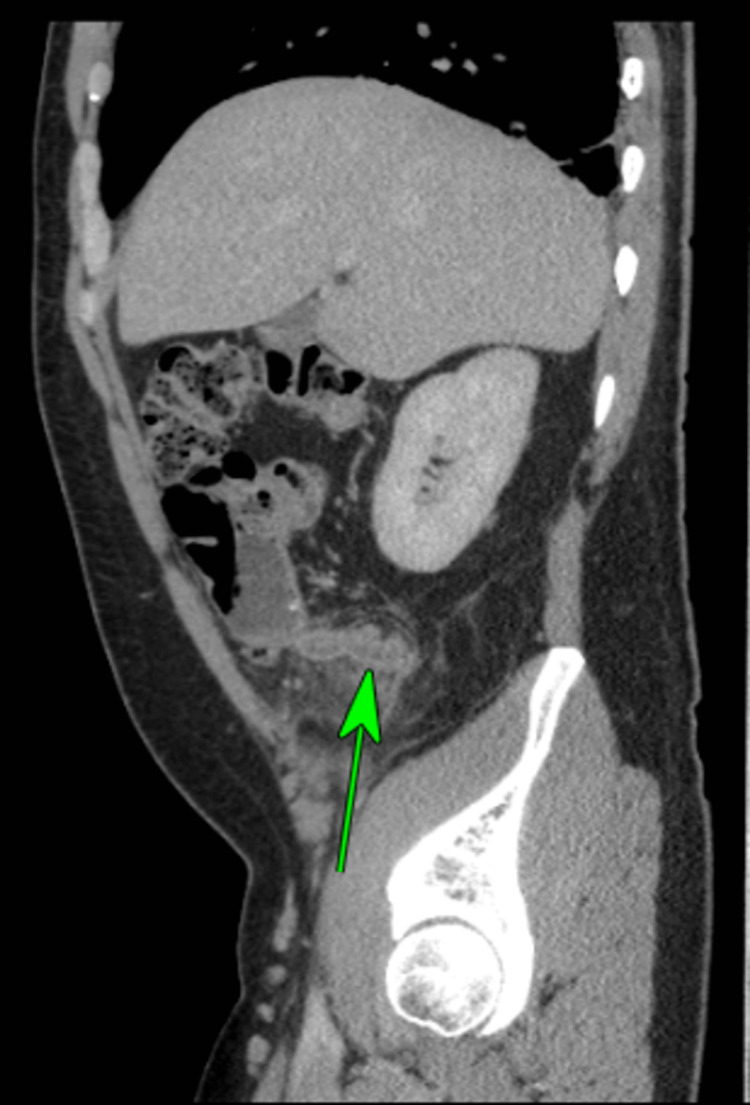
Sagittal CT Scan image of thickened appendix with extensive perifocal fat stranding.
